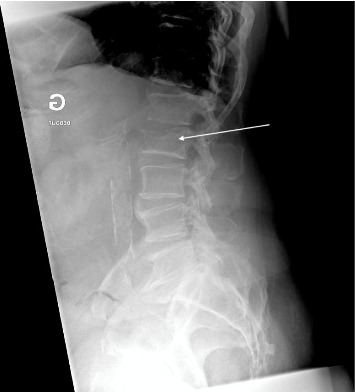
X-ray (03.01) white arrow showing loss of height of L2 with signs of bone destruction.
